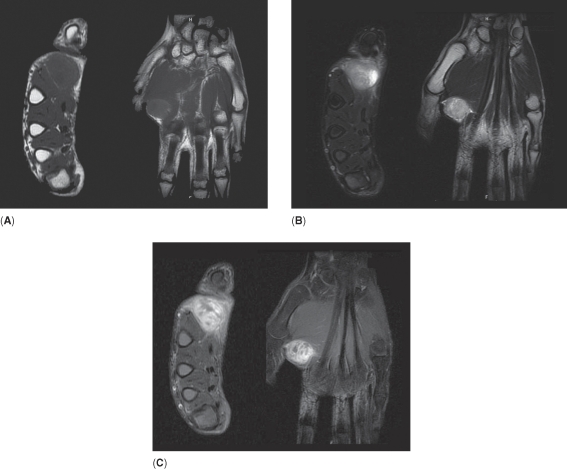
On magnetic resonance imaging (MRI), the mass has low intensity on T1-weighted images (A) and is heterogeneously hyperintense on T2-weighted images (B). After intravenous gadolinium, the mass is enhanced heterogeneously on T1-weighted images (C).
